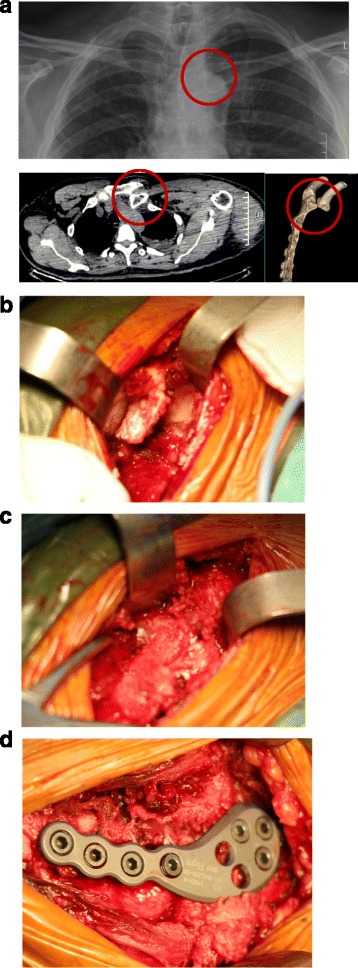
In patient 2, a 31-year-old male, the preoperative x-ray and computed tomography scan a show a posterior dislocation of the sternoclavicular joint. Intraoperative b showing the exposed articular surface of the clavicle from the sternum side; and the position of the medial clavicle was not visible, c showing the medial end of the clavicle being reduced with forceps, and d showing the 3.5-mm locking plate placed anteriorly with three screws in the manubrium and four in the clavicle
